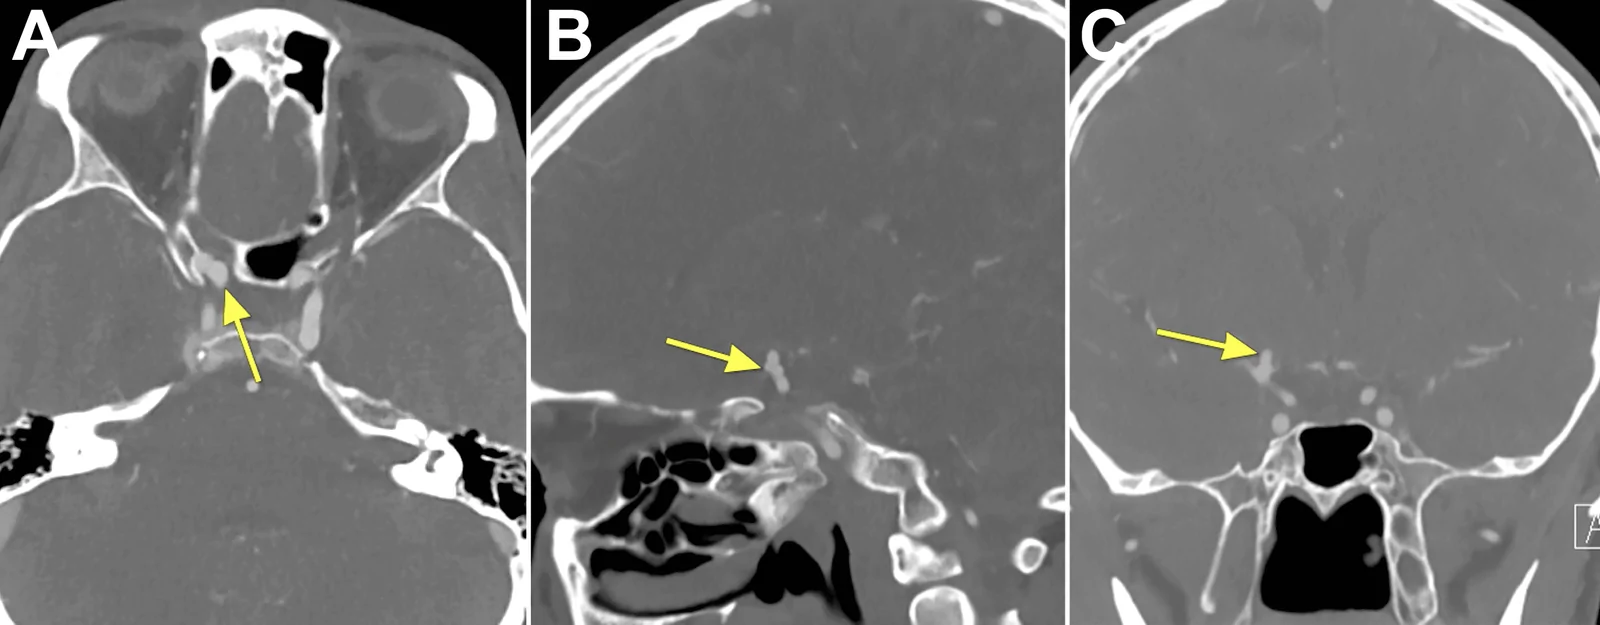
Computed tomography angiography images showing ICA terminus and paraclinoid ICA aneurysms (A) Axial, (B) sagittal, and (C) coronal images demonstrate a 4 × 3-mm anterolaterally projecting aneurysm arising from the right cavernous ICA, as well as a bilobed right carotid terminus aneurysm projecting superiorly and anteriorly, measuring 5 × 3 mm. ICA: internal carotid artery
